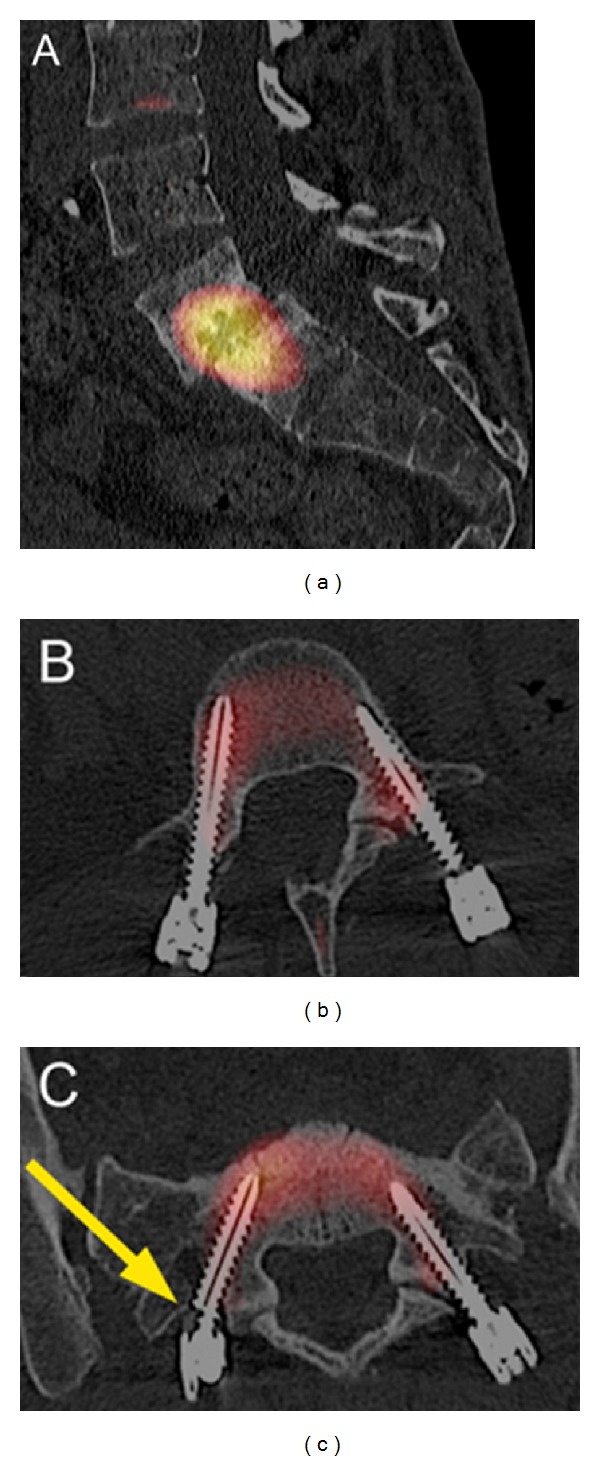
SPECT-CT revealed an intense uptake of the L5-S1 endplates (a) and a fracture of the right S1 screw without uptake and without bone resorption around the screw (c; arrow). The left S1 screw (c) and both L5 screws (b) showed no abnormalities, whereas the rods did.
